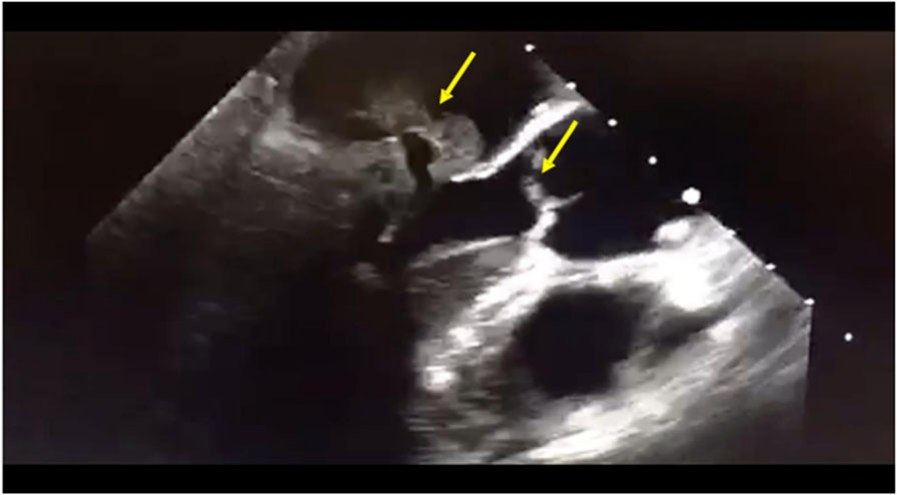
Transesophageal echocardiography (TEE) demonstrating mitral and aortic valvular vegetations
